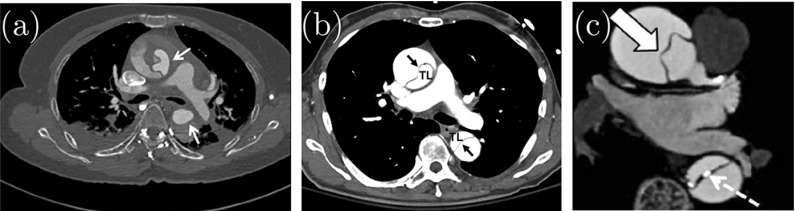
CT scans with buckling of the inner wall in type A aortic dissection: a Fig. 9 in [22], b Fig. 2 in [10], and c Fig. 2 in [23]
